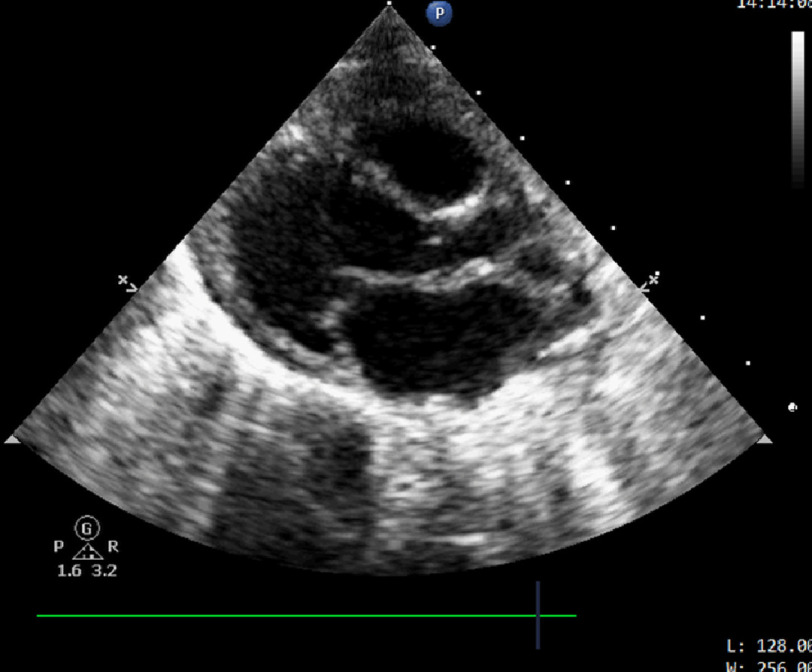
Transthoracic echo, parasternal long axis view shows dilated LV.
